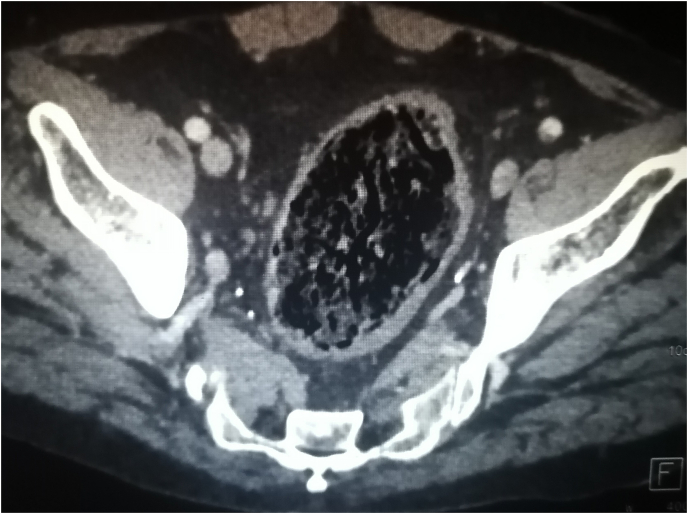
CT scan in the axial plane showing the megarectum after the first surgery.
